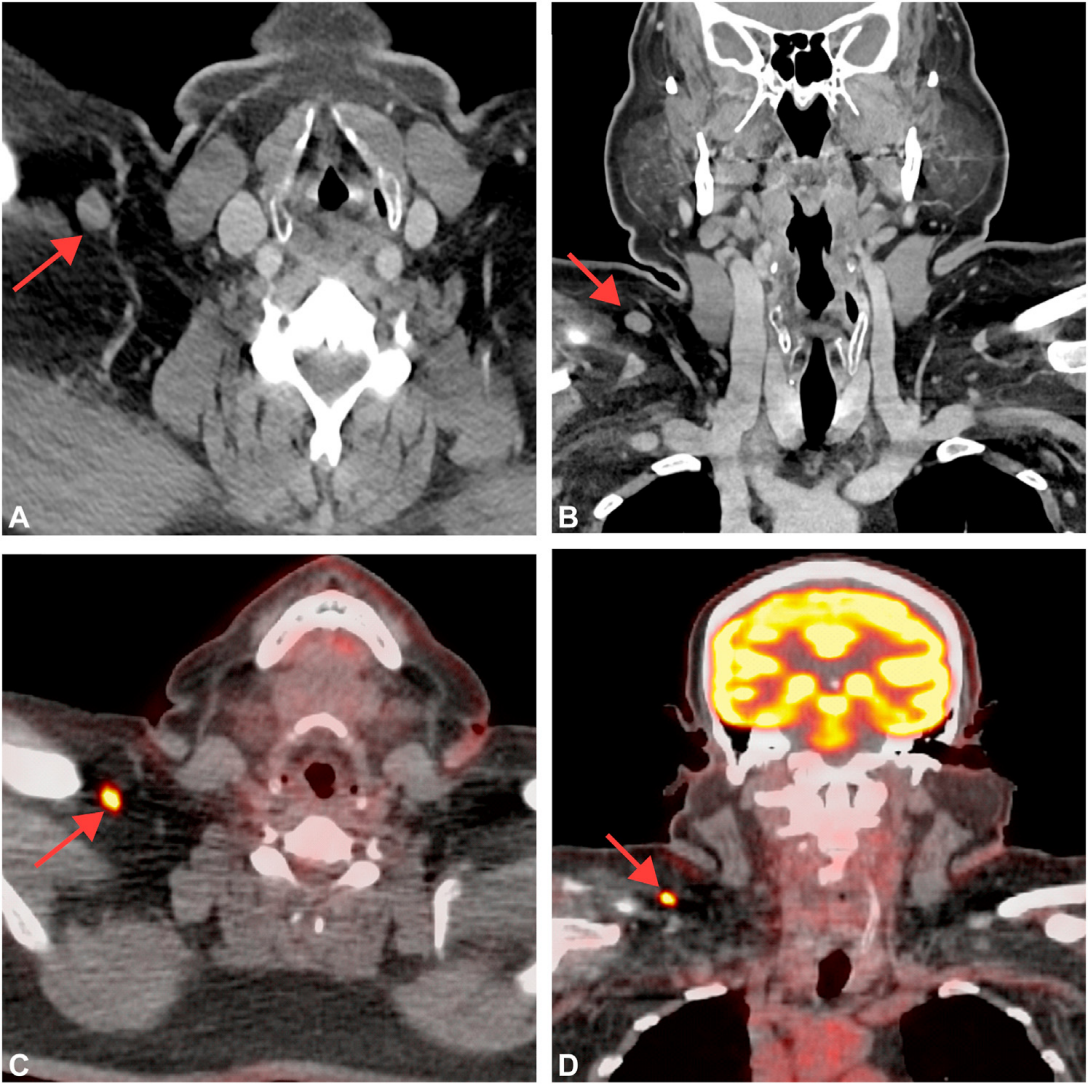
Immediate imaging evaluation of the neck after the biopsy. A and B, Axial (A) and coronal (B) images from the patient's computed tomography (CT) neck with contrast demonstrates an enlarged, approximately 13 mm short axis right supraclavicular lymph node (*red arrows*), suspicious for nodal metastatic disease; C and D, Axial (C) and coronal (D) images from the patient's PET-CT scan demonstrates F-18 fluorodeoxyglucose (FDG) avidity of the right supraclavicular lymph node with maximal FDG activity measuring 7 Standardized Uptake Value (SUV) (*red arrows*), suggestive of nodal metastatic disease.
